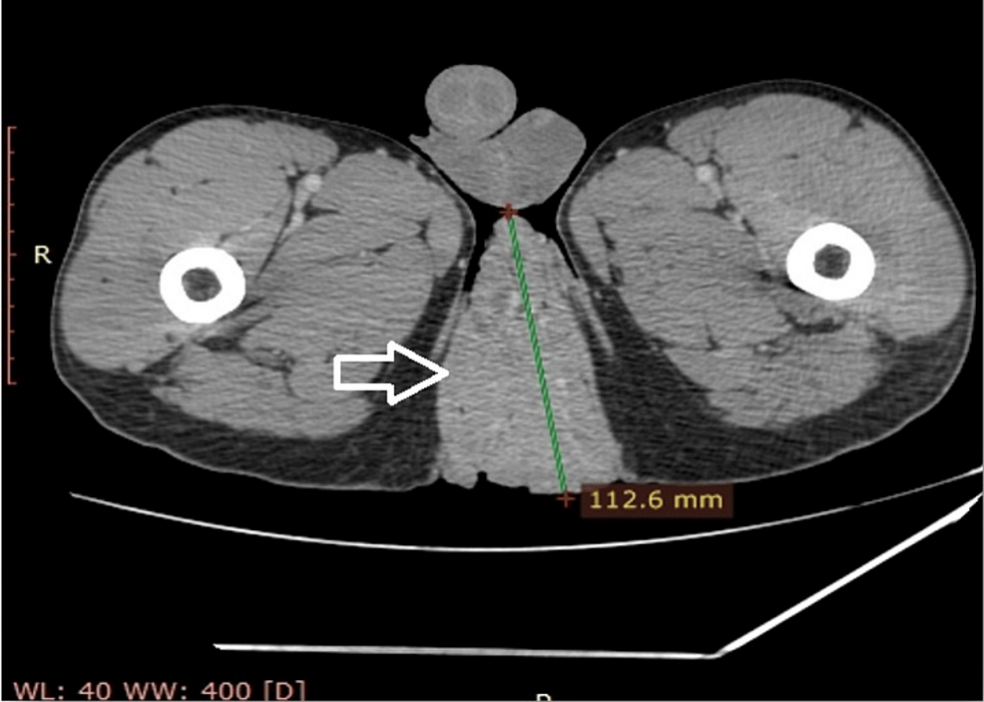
Axial CT scan showing the perineal tumor measuring 112.6 mm (arrow).
